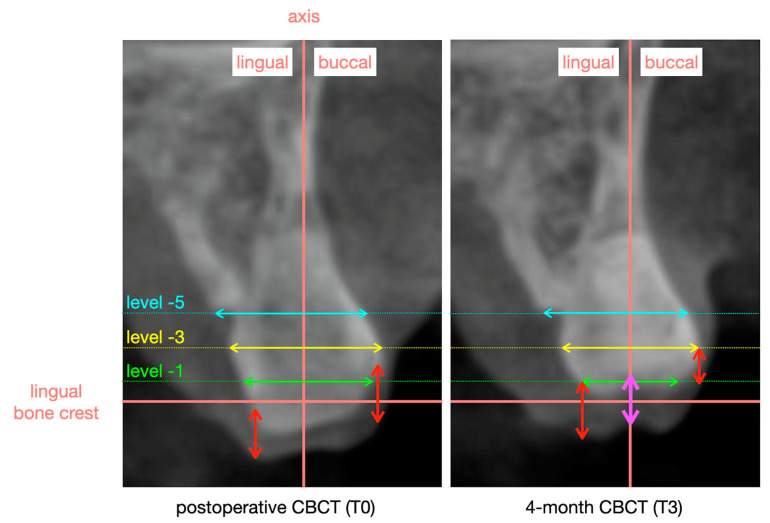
On the 4-month cone-beam computed tomography (CBCT) (T3) at the center of the extracted tooth, the following reference lines were established: the long axis of the extracted tooth, the lingual bone crest level perpendicular to the extracted tooth’s long axis, level −1 mm (green), level −3 mm (yellow) and level −5 mm (cyan) apical to the lingual bone crest and perpendicular to the extracted tooth’s long axis. Soft tissue thickness in the center of the site was measured along the vertical reference line of the extracted tooth’ long axis as the vertical distance between the alveolar process and the soft tissue outline (purple arrow). The bone width was registered at the different levels (1 mm level: green arrow, 3 mm level: yellow arrow, and 5 mm level: cyan arrow). The buccal and lingual soft tissue heights were measured as the vertical distance between the alveolar process and the soft tissue outline parallel to the vertical reference line of the extracted tooth’s long axis at the most buccal and lingual part of the alveolar process (red arrows). After all measurements were completed on the 4-month CBCT, the software switched to the superimposed postoperative CBCT (T0) image, maintaining all reference lines so that the same measurements could be performed.
